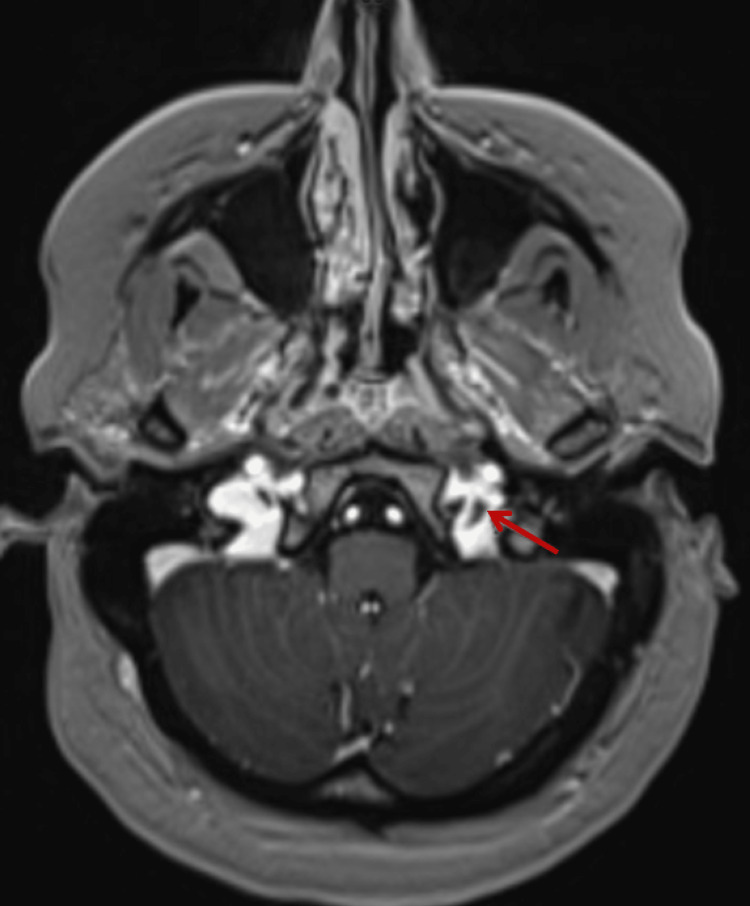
Axial MRI of the head and neck demonstrating a filling defect in the left jugular bulb (red arrow)
